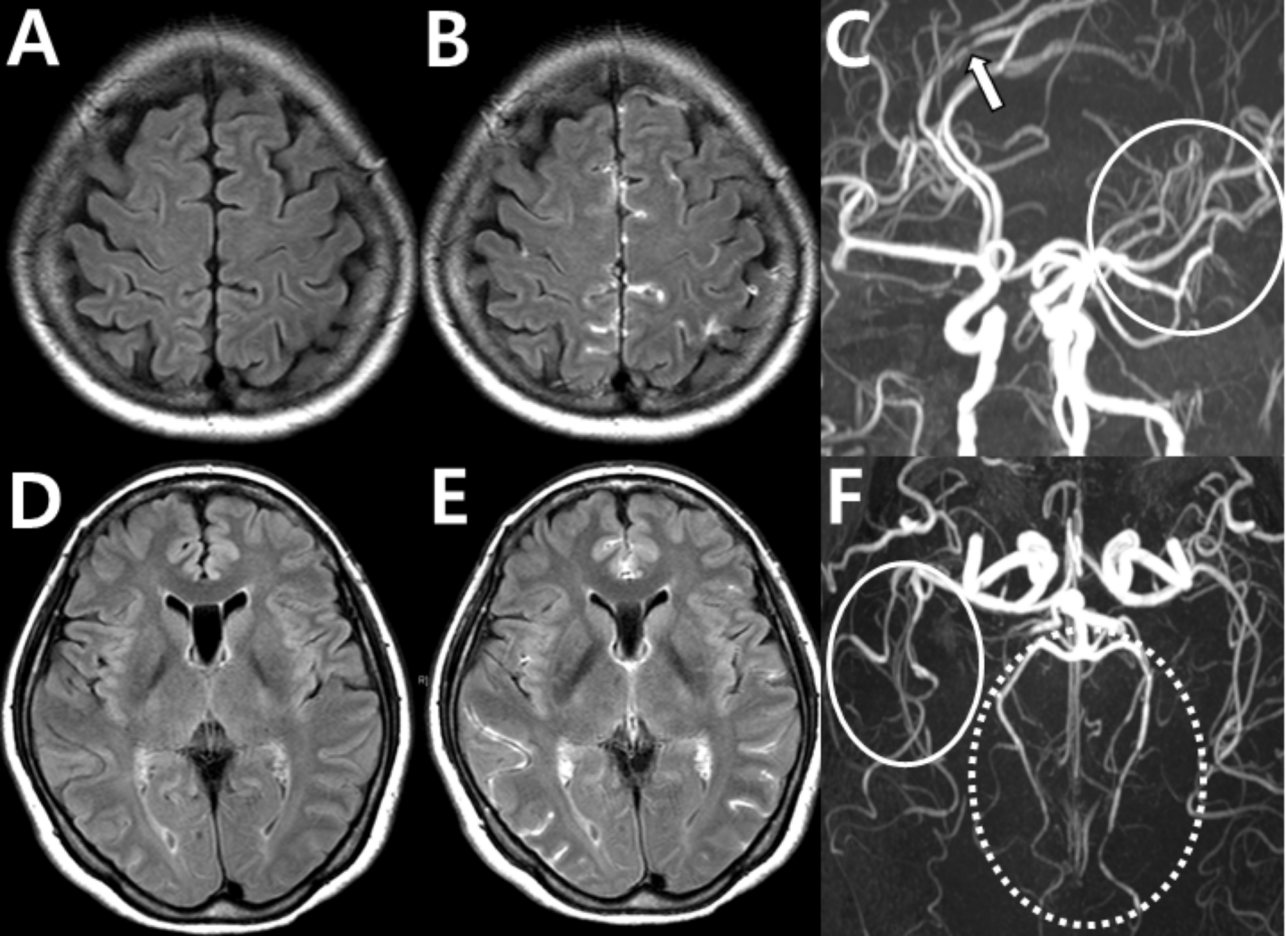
Blood-brain barrier disruption in reversible cerebral vasoconstriction syndrome. BBB breakdown is observed using contrast-enhanced FLAIR imaging in a 56-year-old female with RCVS (case 2). (A, D) FLAIR images without contrast. (B, E) The same sections with contrast-enhanced FLAIR MRI show BBB disruption through hyperintense CSF. (C, F) MRA shows typical multifocal steno-dilatation in the ACA (arrow), left MCA (solid circles), and distal PCAs (dotted circles)
